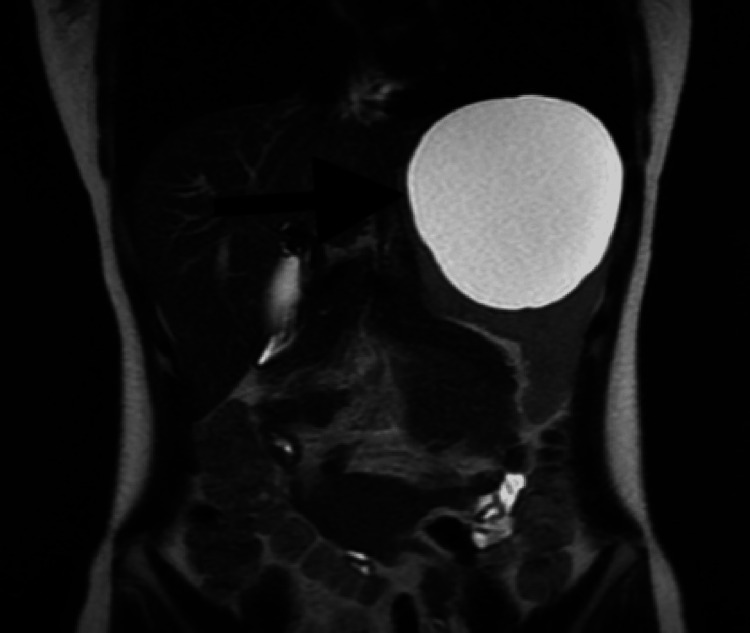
Magnetic resonance imaging of the abdomen/pelvis with and without contrast: a large splenic cyst can be seen within the left upper quadrant exerting a mass effect on the stomach.
